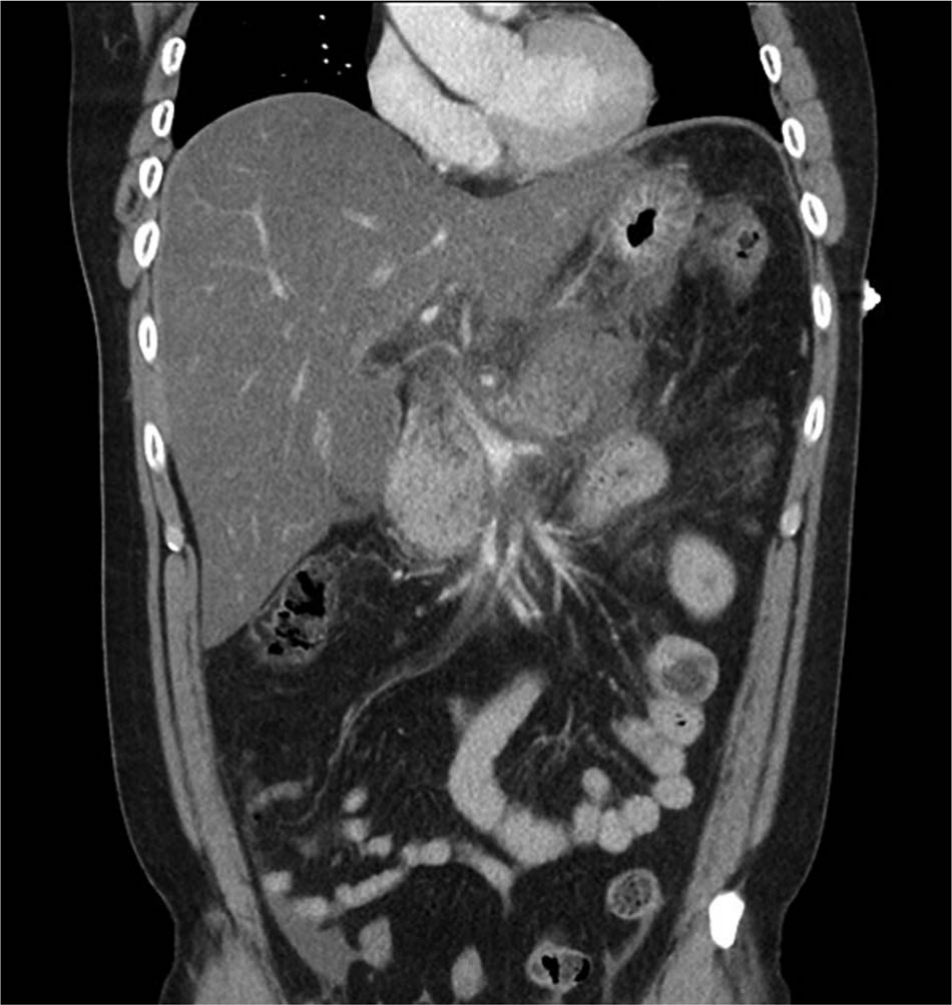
Coronal CT image demonstrating body and tail pancreatitis with potential early signs of necrosis.
